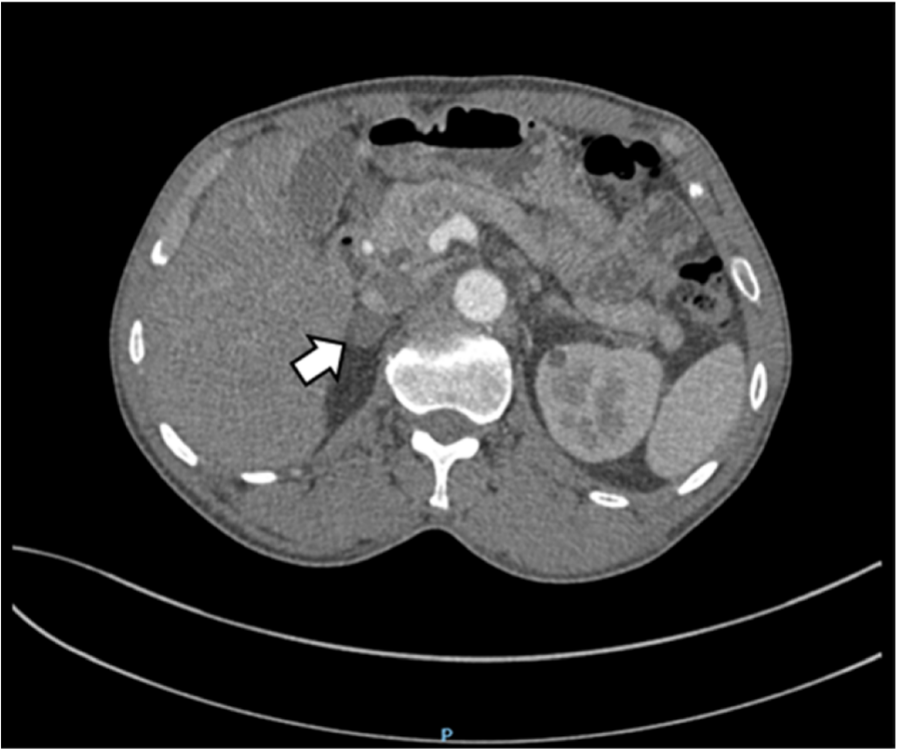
Contrast computed tomography scan shows mass in the right adrenal gland. Arrow: right adrenal tumor
